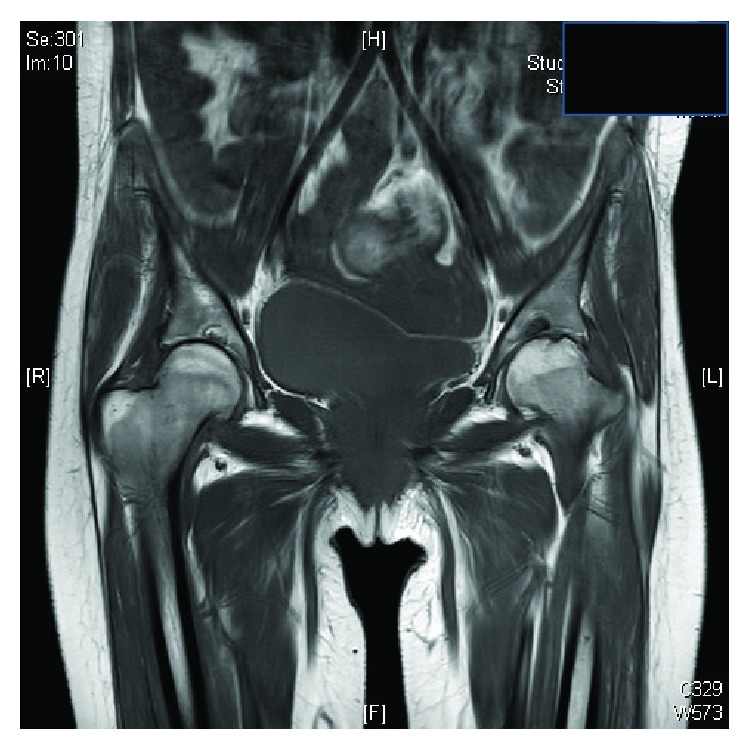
MRI of the hip at age 19. Appearances are in keeping with inflammatory arthropathy.
